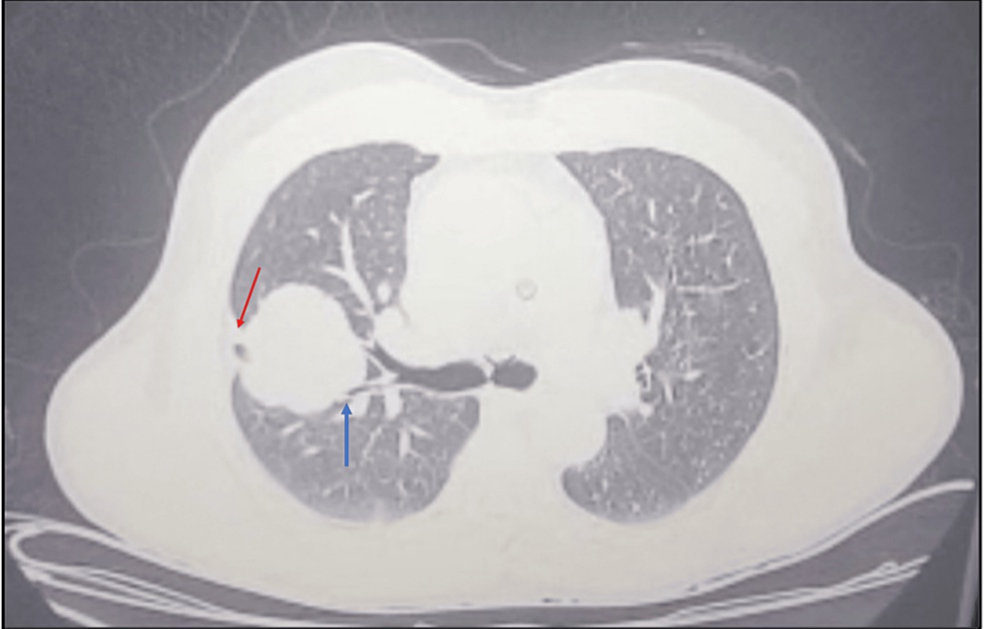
Chest HRCT (lung window) showing bronchus cut-off sign
